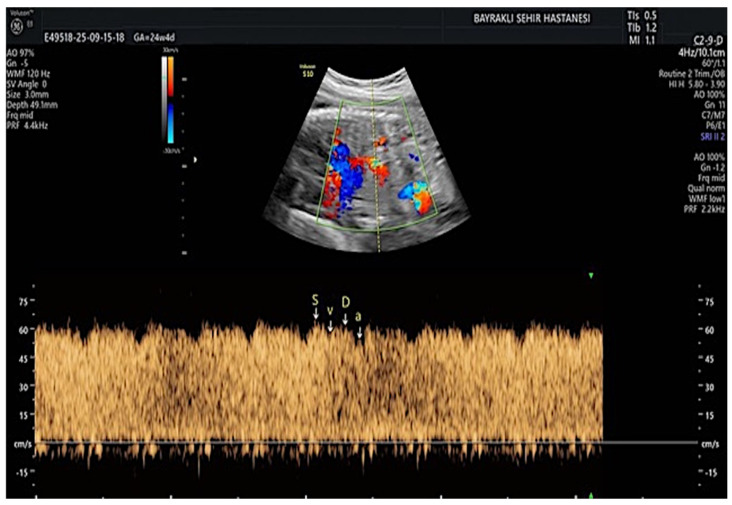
Spectral Doppler waveform of the ductus venosus demonstrating S−, D−, v−, and a−wave velocities.
